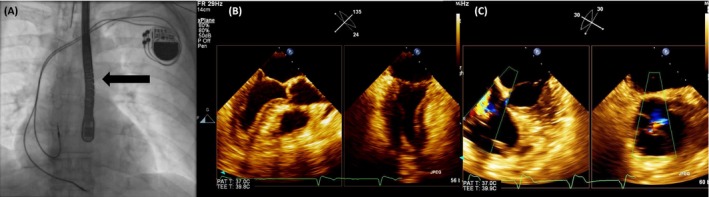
Postimplantation of the new leads. A, Position of the new leads. The static echocardiography probe is also visible in mid‐esophageal position (arrow); B, biplane image of the left cavities; C, biplane image of the tricuspid valve (left long axis, right short axis), displaying a stable pre‐existent tricuspid valve regurgitation. Note that both the left and the right cavities could be imaged in multiplane, by remotely manipulating the field of view, without altering the probe position
